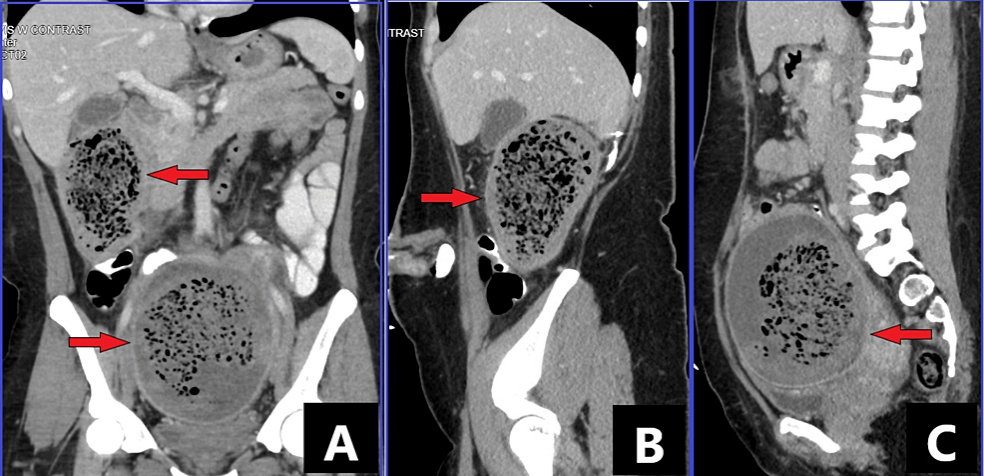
Peripherally enhancing, contained, heterogenous areas with both hypodense and fluid components (arrows) seen on CT scan of the abdomen and pelvis in the coronal (A) and sagittal view (B, C).
